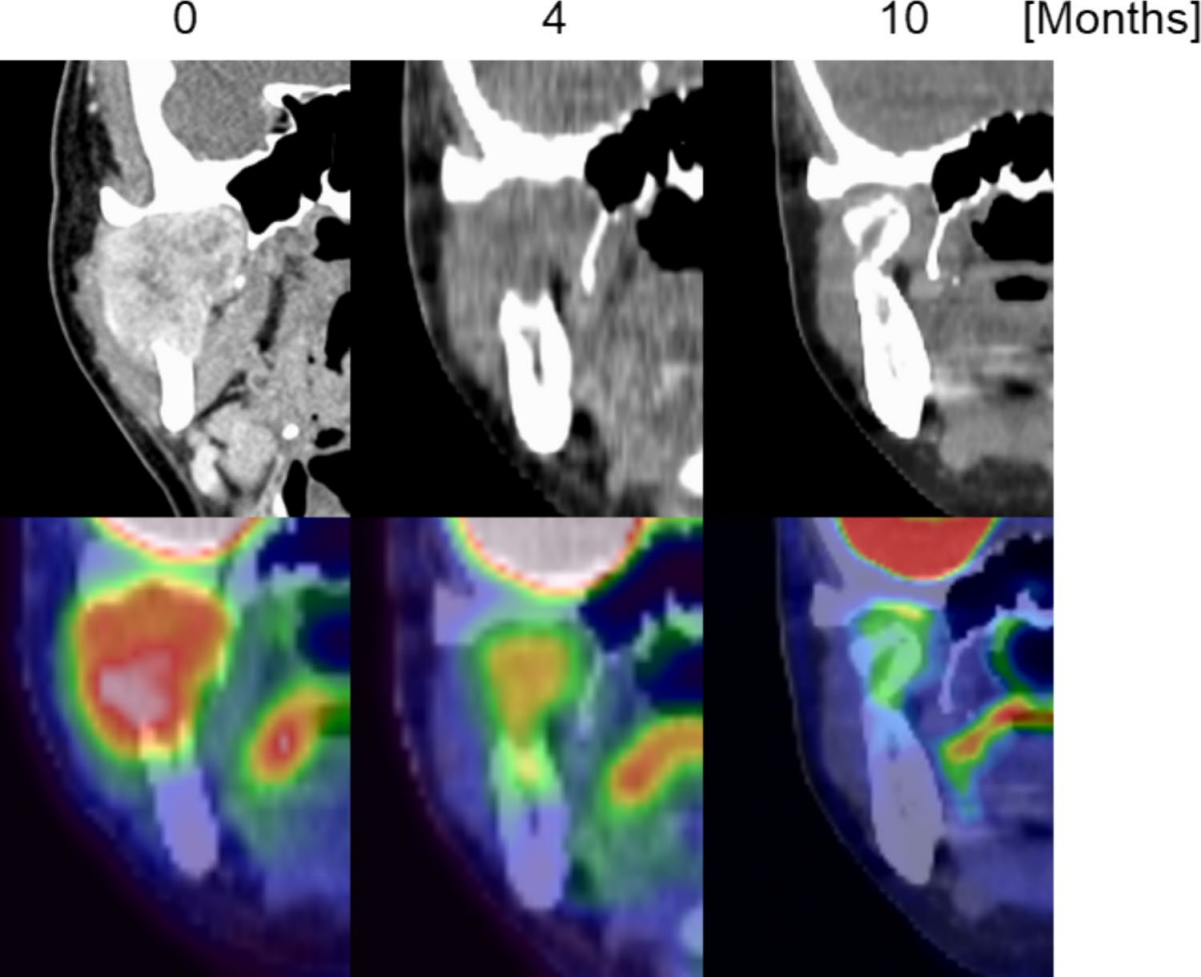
PET/CT images of a patient with bone metastasis to the right condyle at baseline (0 months), 4 months, and 10 months post-chemotherapy. At 0 months, abnormal fluorodeoxyglucose F18 (^18^F-FDG) standardized uptake value maximum (SUVmax = 4.7) was observed in the right condyle. At 4 months, CT did not show bone remineralization but ^18^F-FDG uptake persisted (SUVmax = 3.4). At 10 months, bone remineralization was visible at the same site with corresponding morphology
